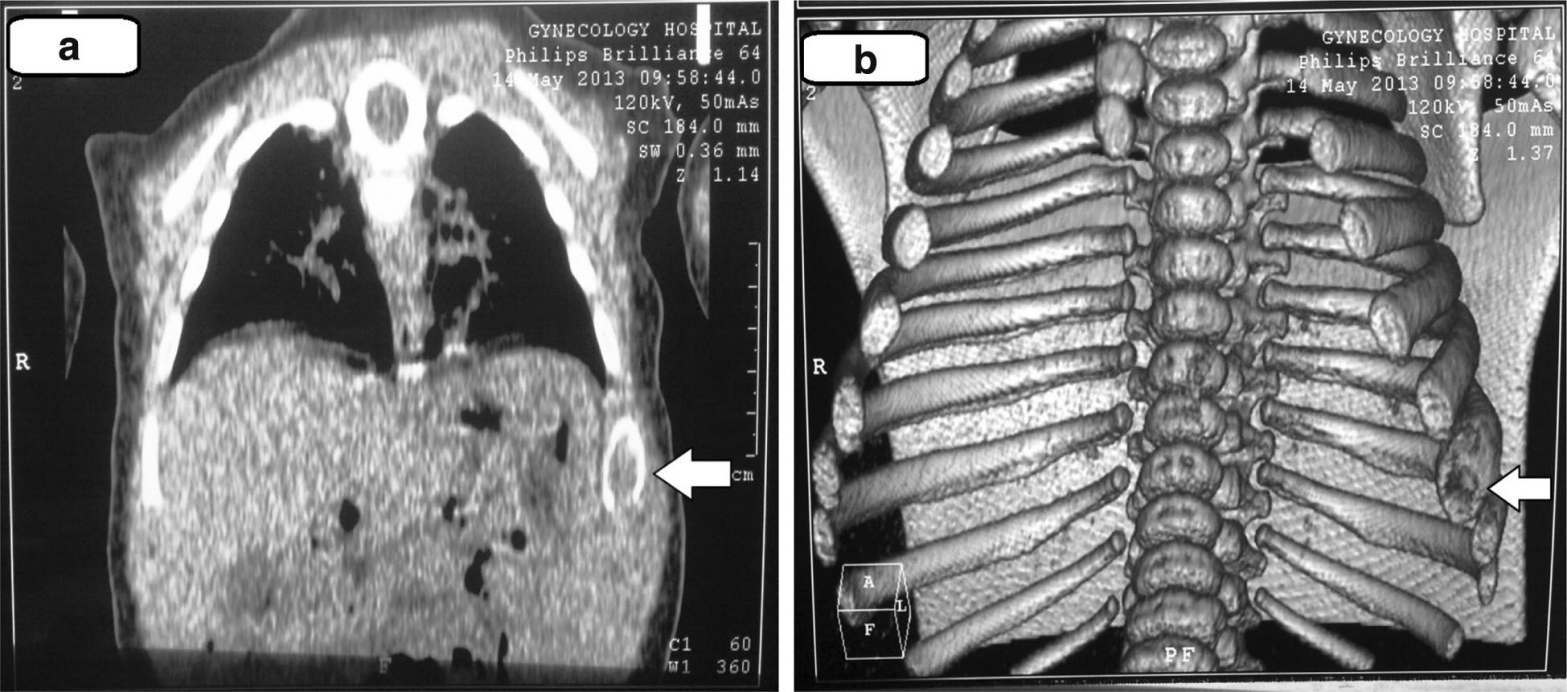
Chest computed tomography native (a), three dimensional (b), showed the lateral part of the left 8th ribs with expansile lytic changes with breaching out and some surrounding soft tissue components.
